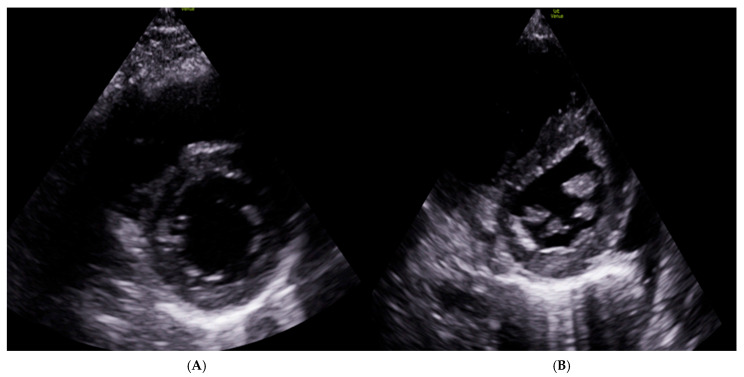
Parasternal short axis view; (A) normal morphology of the interventricular septum; (B) D-sign in the context of acute pulmonary embolism (image courtesy of E. Polyzogopoulou).
